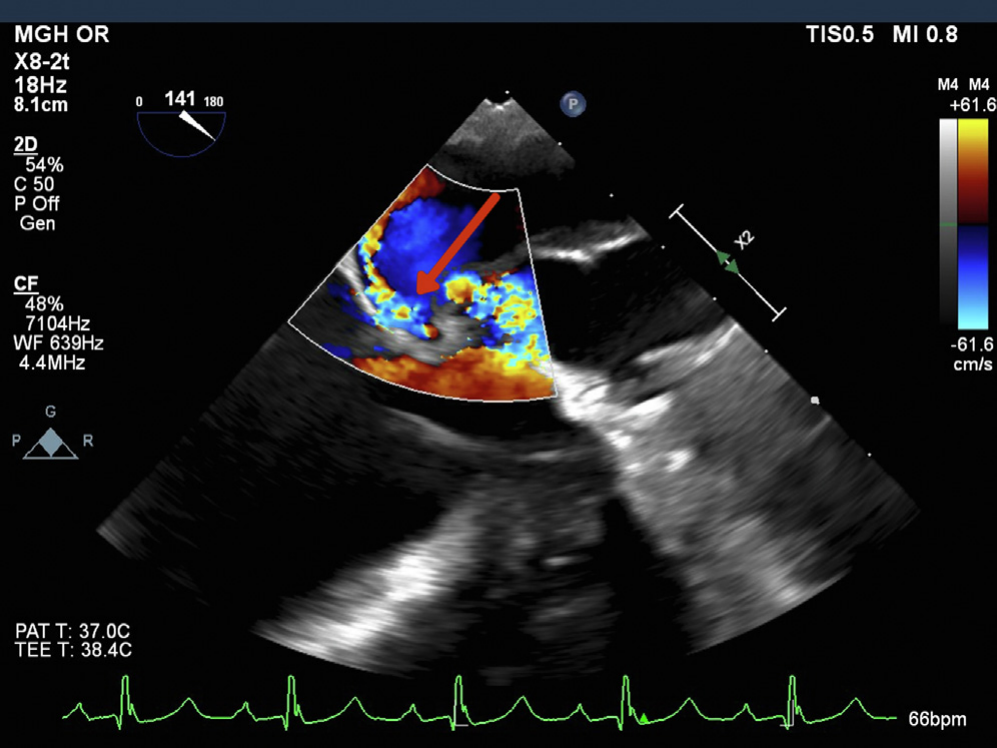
Transesophageal Echocardiogram Demonstrating Systolic Blood Flow via Pseudoaneurysm to Left Atrium Transesophageal echocardiogram demonstrating systolic blood flow from the left ventricular outflow tract, through the pseudoaneurysm, and into the left atrium along the anterior mitral leaflet. The red arrow marks the site of the pseudoaneurysm.
